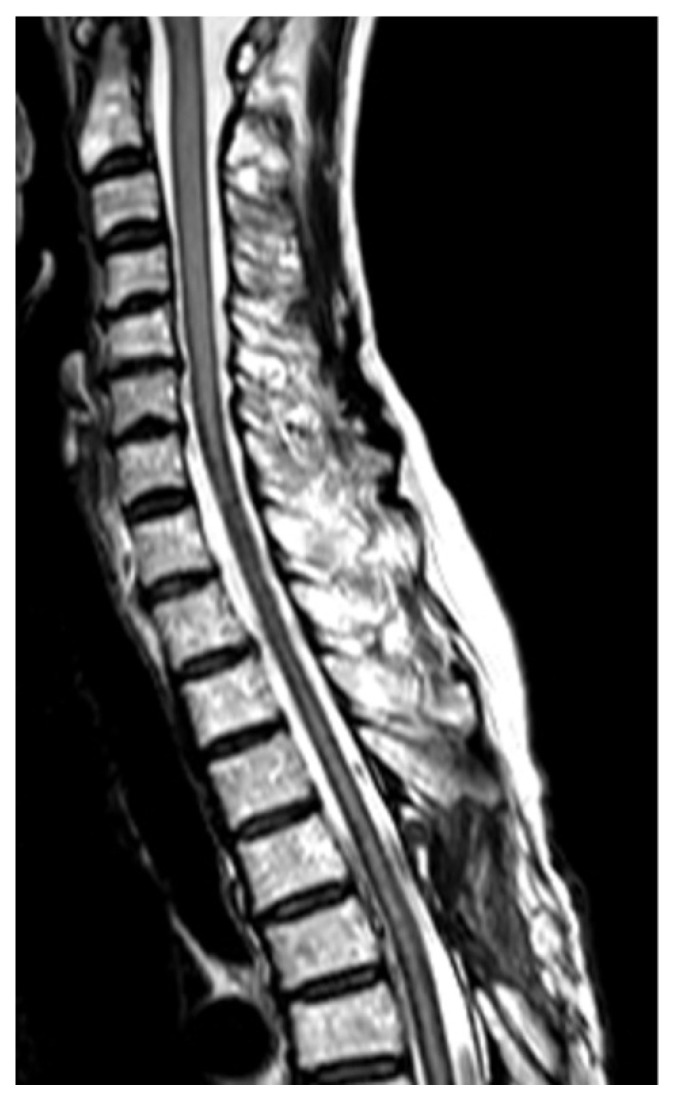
T2-weighted MRI of the cervical to upper thoracic spine taken three months after surgery confirms the resolution of the anterior epidural fluid collection.
